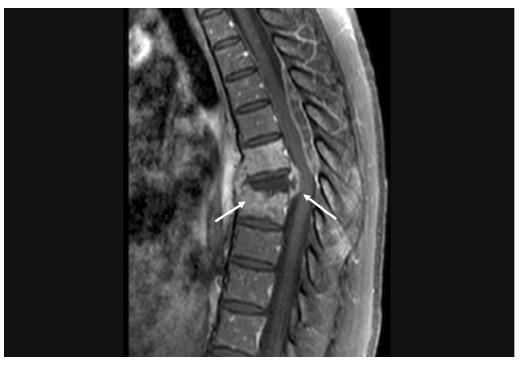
Magnetic resonance imaging. Sagittal contrast-enhanced T1-weighted fat-suppressed MR weighted image of the thoracic spine showing paravertebral collections with peripheral enhancement, enhancement of the T6 and T8 vertebral bodies (arrow), a necrotic collapsed T7 vertebral body, and a posterior epidural mass with peripheral enhancement at the T4-T7 levels, suggesting epidural abscess. An anterior epidural collection at the T7 level combined with angular kyphosis resulted in spinal cord compression (arrow).
